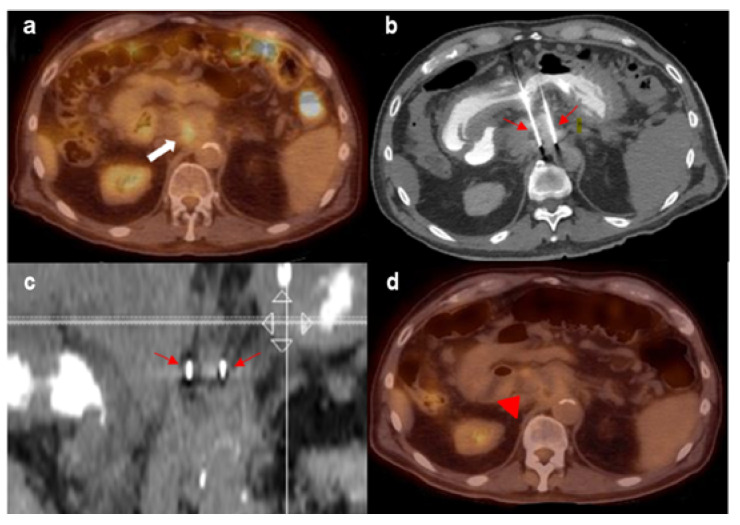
(a) FDG-avid recurrent pancreatic mass (white thick arrow). (b,c) Two IRE probes in place bracketing the mass (thin red arrows). (d) No residual FDG avidity post-treatment (red arrow).
